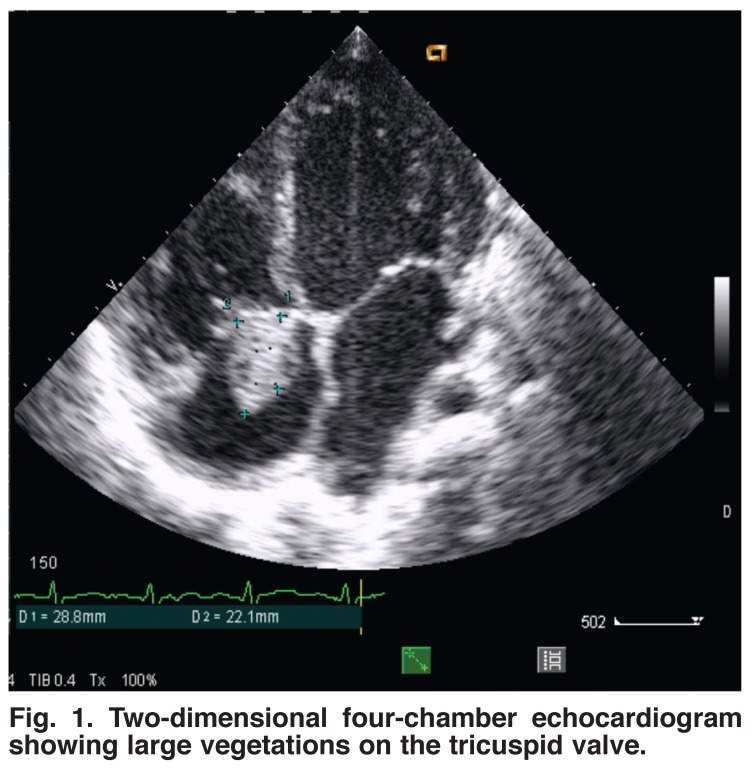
Two-dimensional four-chamber echocardiogram showing large vegetations on the tricuspid valve.
